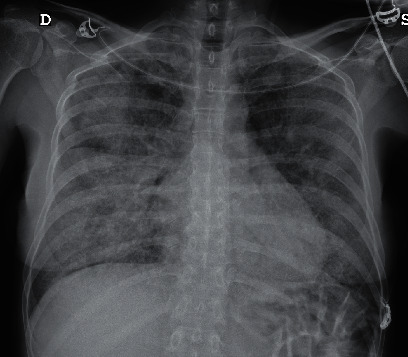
Chest X-ray on admission. Note the bilateral interstitial pattern with bilateral diffuse lung involvement.
